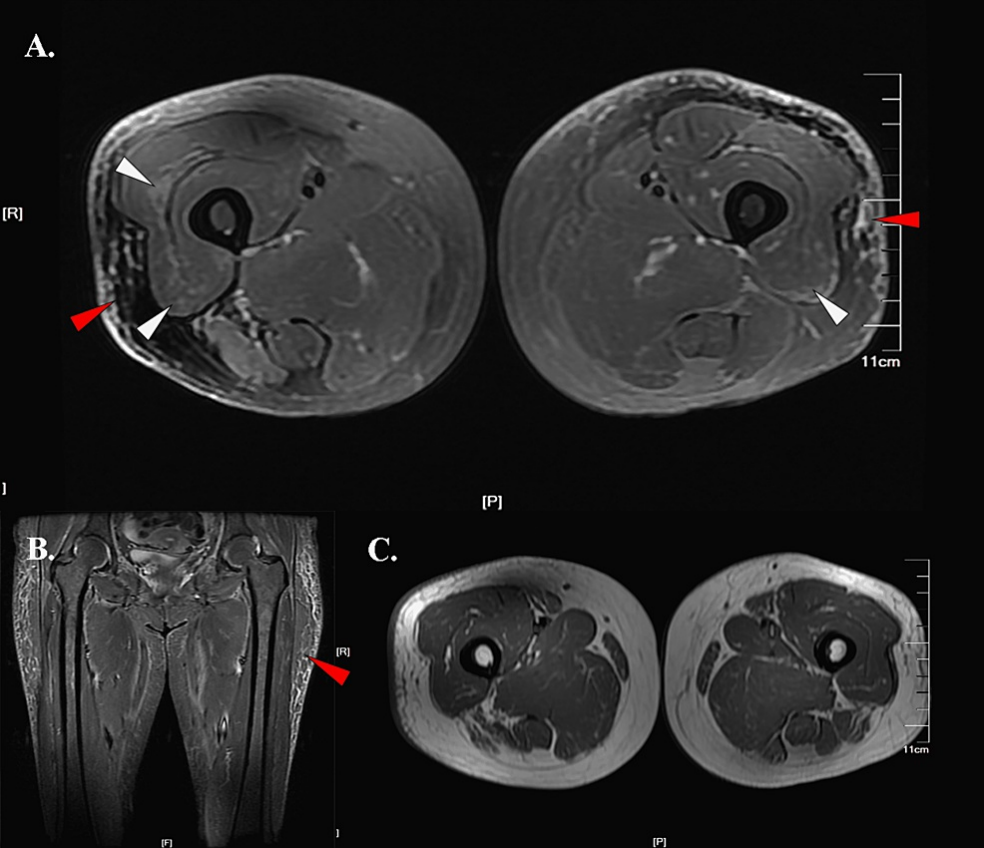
Thigh muscle MRI images of Case 1 A. An axial T2 fs sequence image showed subcutaneous adipose tissue involvement (red arrows) and peripheral honeycomb edema signal of the vastus lateralis (white arrows). B. A coronal STIR sequence image showed subcutaneous adipose tissue involvement (red arrow). C. An axial T1 sequence image showed minimum fatty infiltration. Abbreviations: T2 fs, T2-weighted fat saturation; STIR, short time of inversion recovery.
